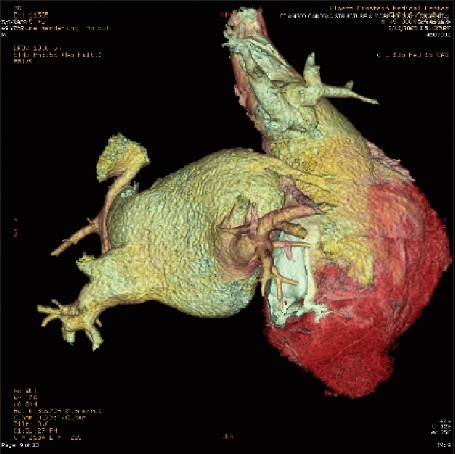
CT reconstruction- showing all four pulmonary veins. Right superior pulmonary veins can be seen draining in to superior vena cava
